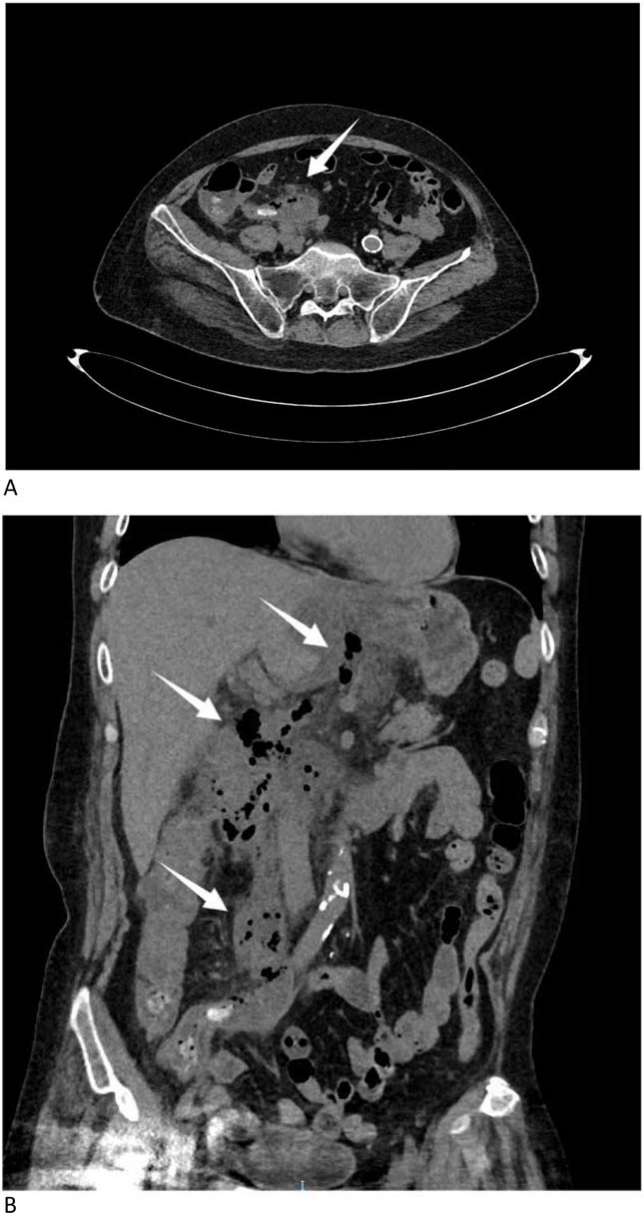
(A) CT scan identified an appendicolith with diffuse infiltration (arrow). (B) CT scan showed a large amount of gas in the retroperitoneal space, extending from the cecum along the mesentery to the stomach (arrows).
